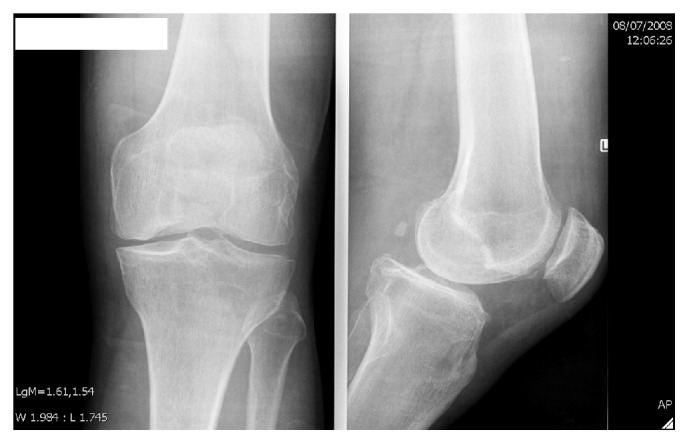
Anteroposterior and profile view X-rays of the knee.
